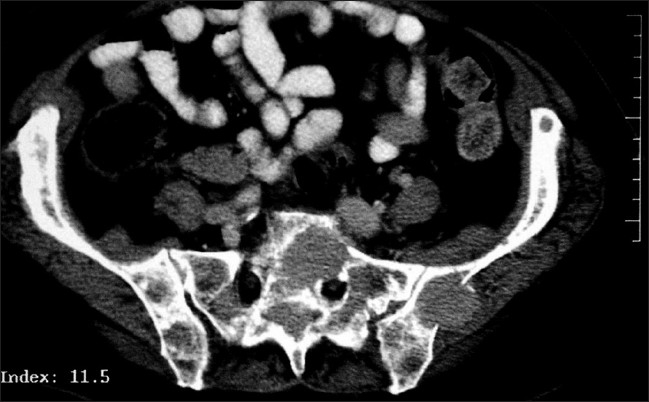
CT scan showing soft tissue infiltration into the left sacrum
